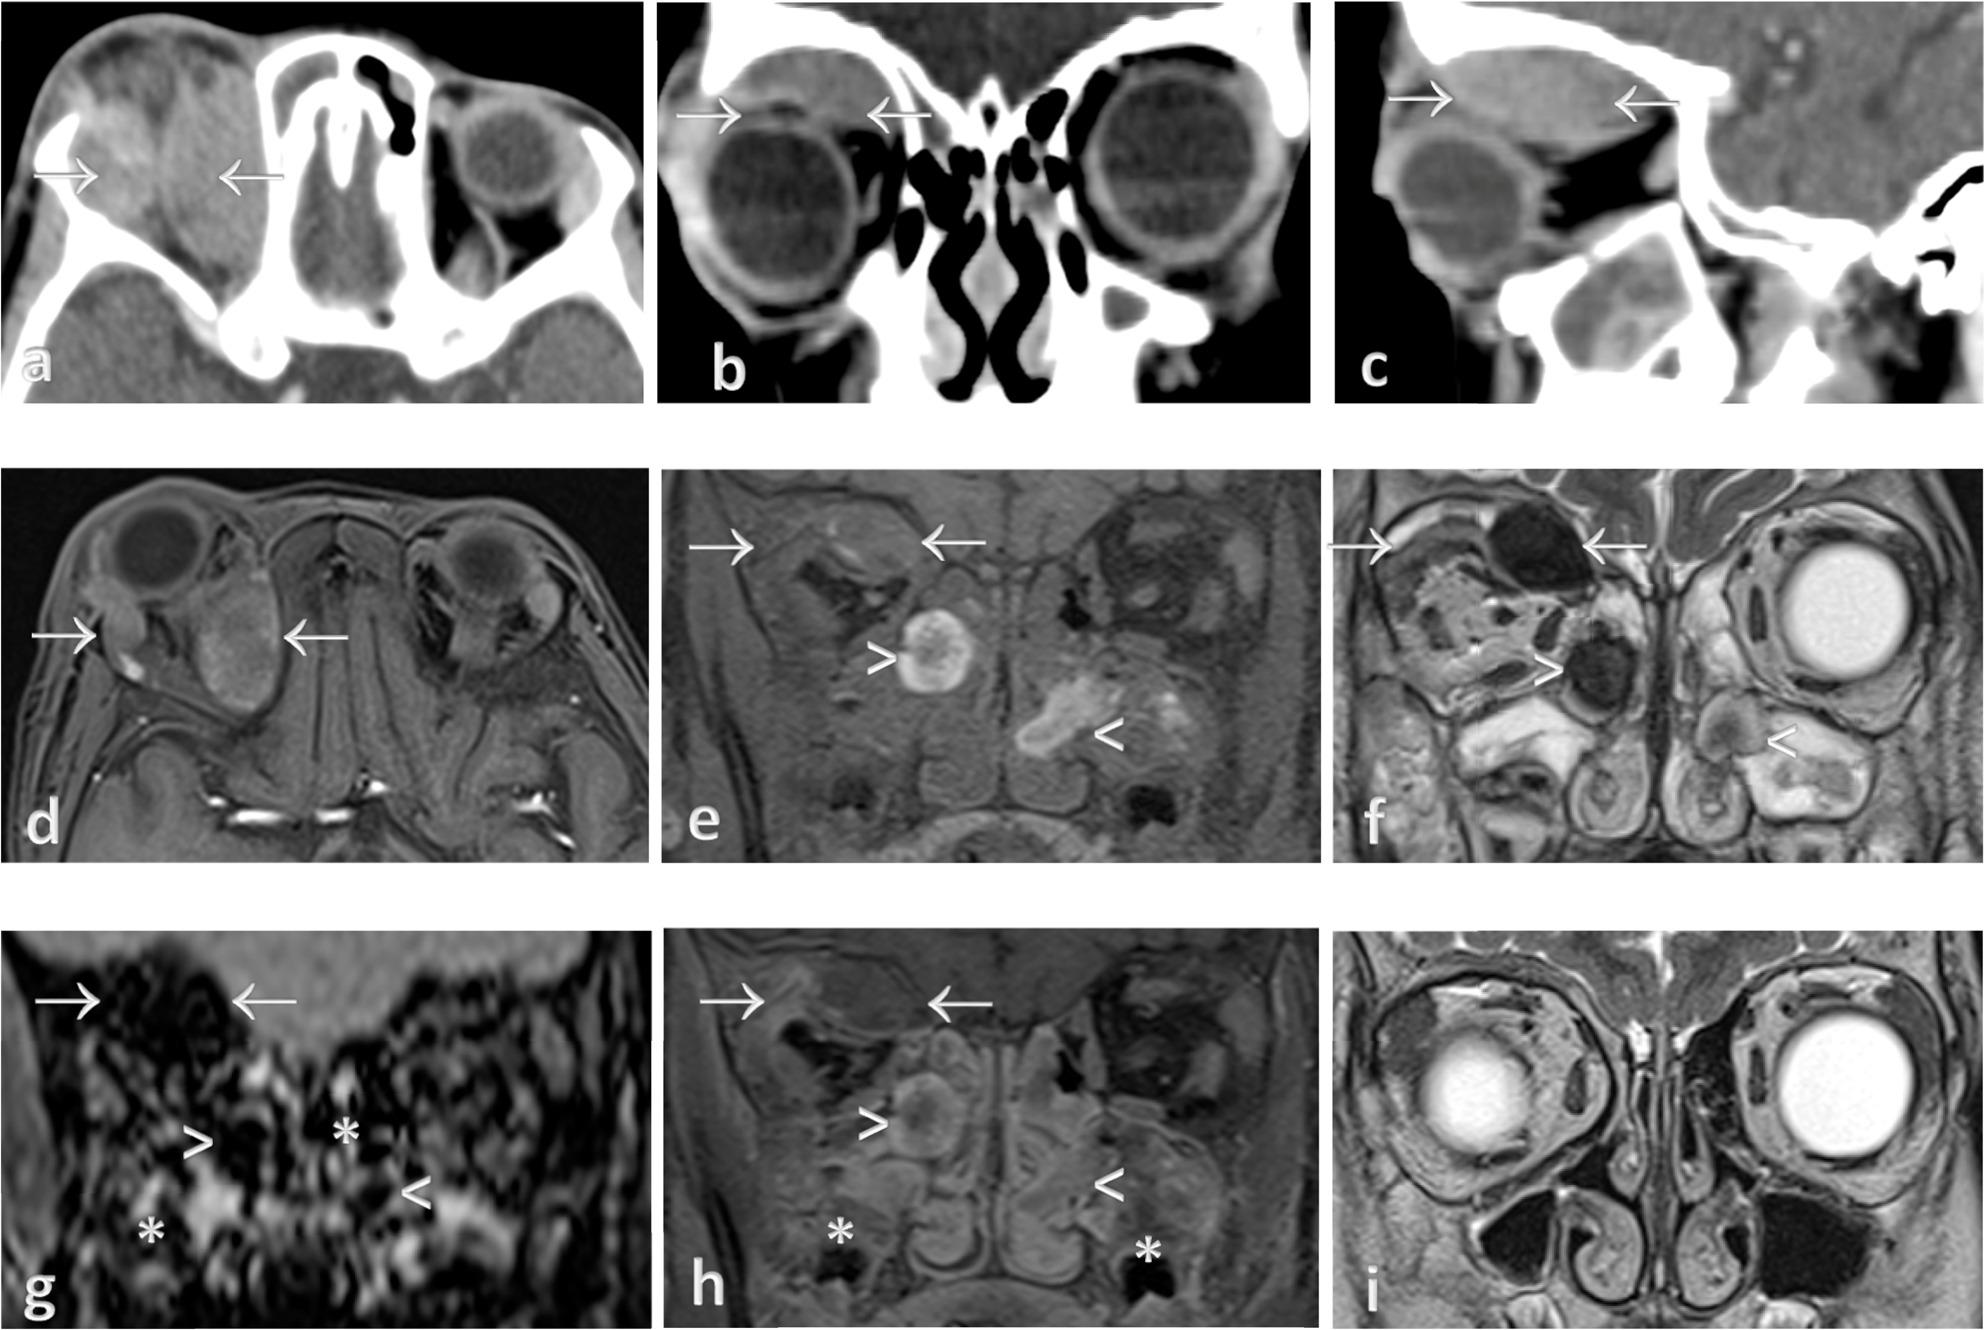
CT and MRI orbit of case 1. a, b, c Contrast-enhanced CT Orbit in axial, coronal, and sagittal images show rim-enhancing collections (arrow) at superior aspect of right orbital roof, extending to the medial and lateral orbital walls. d, e, f T1 fat -suppressed axial and coronal, T2w coronal images of the orbit show T1w isointense and mixed T2w hypointense to hyperintense collections at right orbital roof (arrow), with T1w heterogeneously hyperintense and T2w hypointense collections at bilateral nasal meati (arrow head). g SWI coronal image shows blooming artifacts at right orbital (arrow) and bilateral nasal meati (arrow head), and bilateral maxillary sinuses (asterisk). h T1 fat-suppressed post-contrast coronal image shows rim-enhancing collection at right orbit (arrow) with heterogeneously enhancing intranasal (arrow head) and intrasinal regions (asterisk). i T2w coronal image (6 months later) shows resolution of the collections
